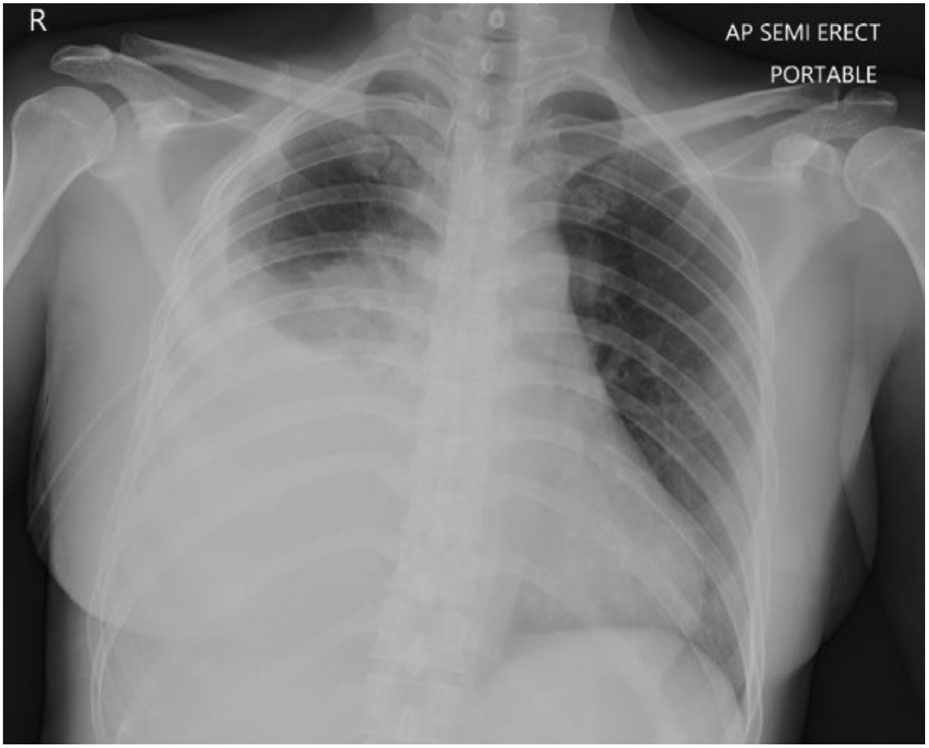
Chest radiograph during initial presentation showing a right lower zone opacity due to lung consolidation and surrounding pleural effusion.
